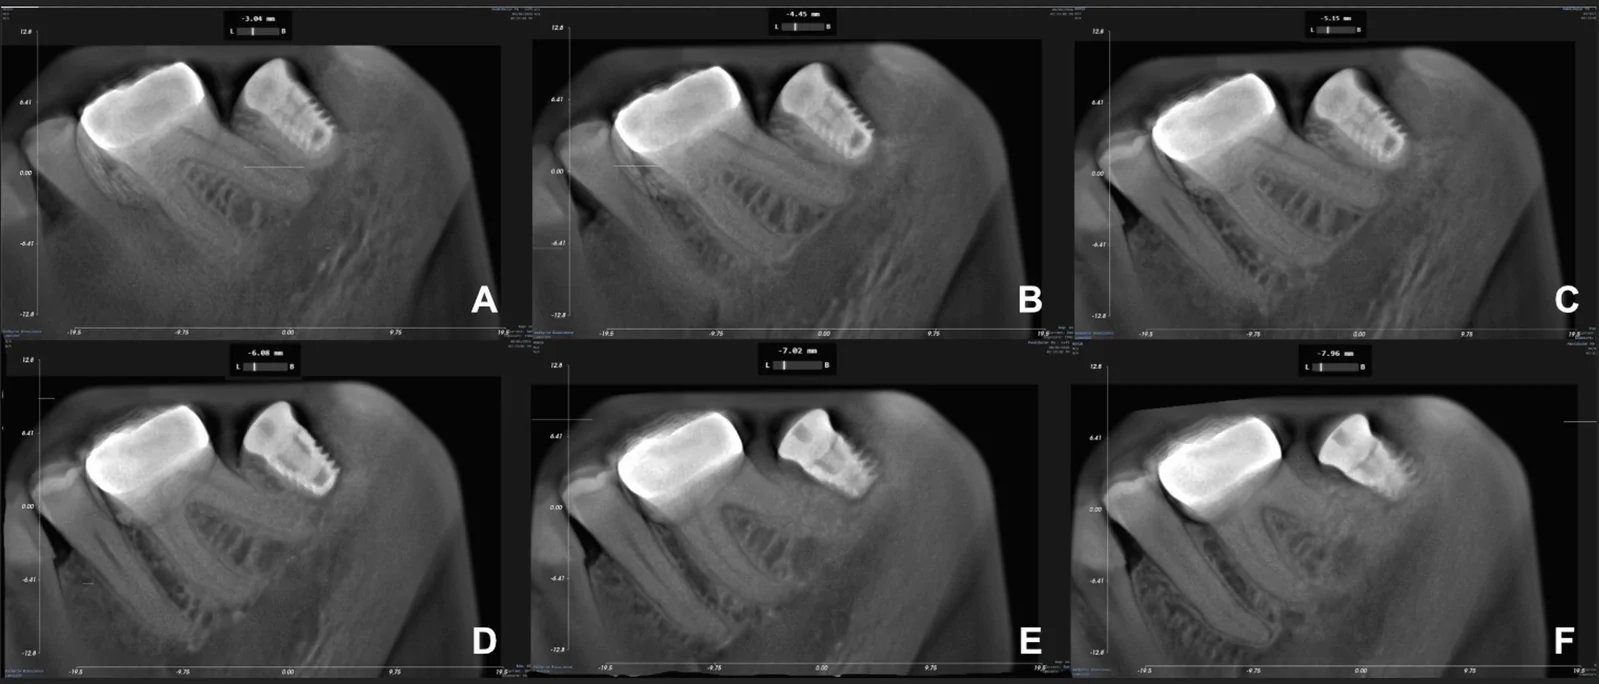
Sequential cross-sectional tomosynthesis images of the posterior mandibular implant site obtained with the Lumos 3DX. The numerical values displayed on the images identify the relative position of the reconstructed sections within the tomosynthetic dataset. Viewing the sequential sections allows assessment of implant position in the buccolingual dimension and demonstrates its relationship to the surrounding cortical bone and inferior alveolar canal, information that cannot be directly determined from the conventional two-dimensional periapical image.
